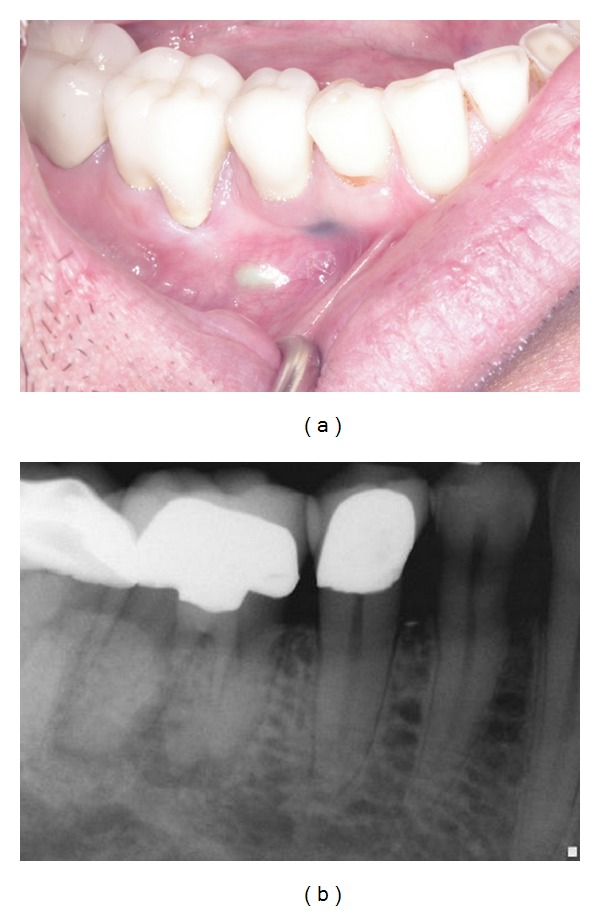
(a) 5 mm by 3 mm yellowish-white mass in the mucobuccal fold region of the lower right second premolar. A bluish-purple hyperpigmentation similar to the appearance of an amalgam tattoo is also noted on the alveolar process between the premolars. (b) Radiograph shows no radiopacity in the region of the premolars. The lingual tori can be seen as well-defined areas of radiopacity overlying the tooth roots of the molars.
